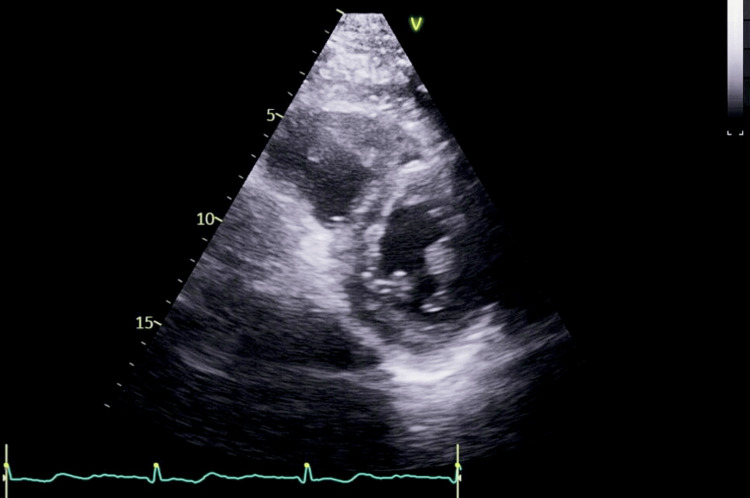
Parasternal short-axis view acquired via transthoracic echocardiography, visualizing the patient's cardiac anatomy
